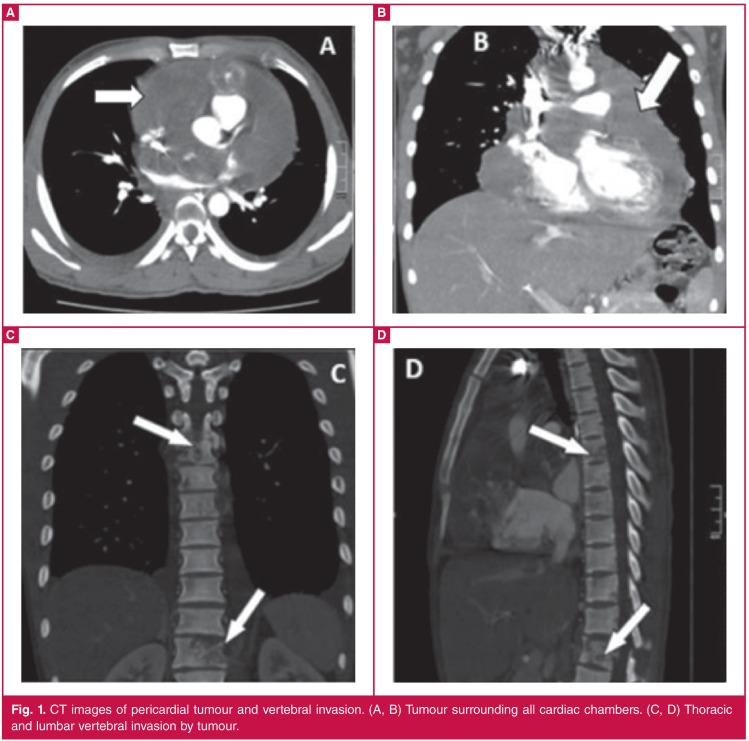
CT images of pericardial tumour and vertebral invasion. (A, B) Tumour surrounding all cardiac chambers. (C, D) Thoracic and lumbar vertebral invasion by tumour.
